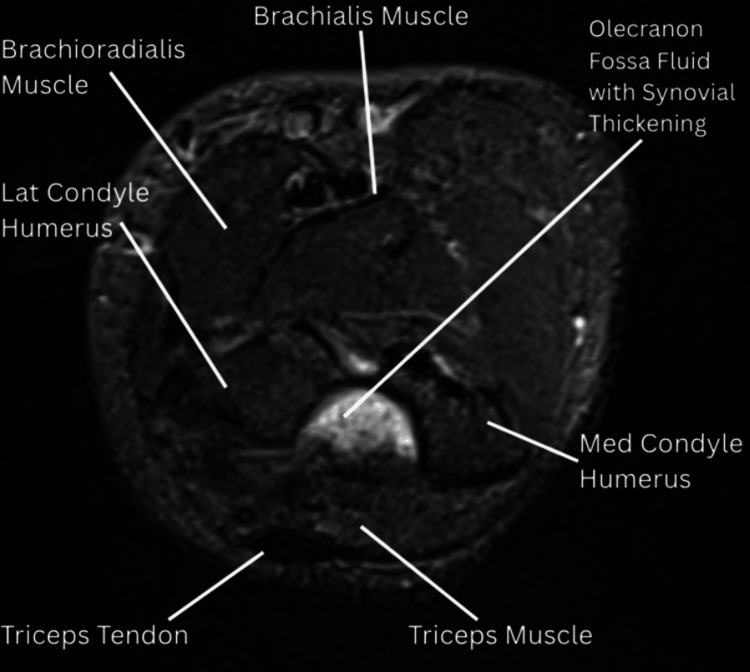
MRI (pre-treatment): axial proton density fat-suppressed (PDFS) image demonstrating mild joint effusion in the olecranon fossa and mild synovial thickening.
